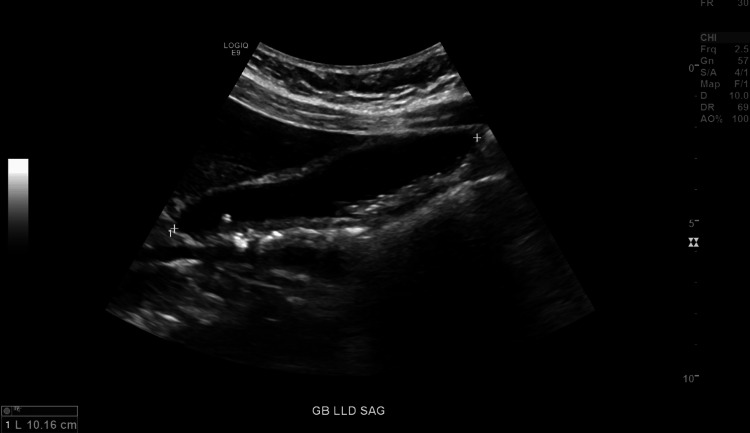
Gallbladder ultrasound with sludge and stone present.
